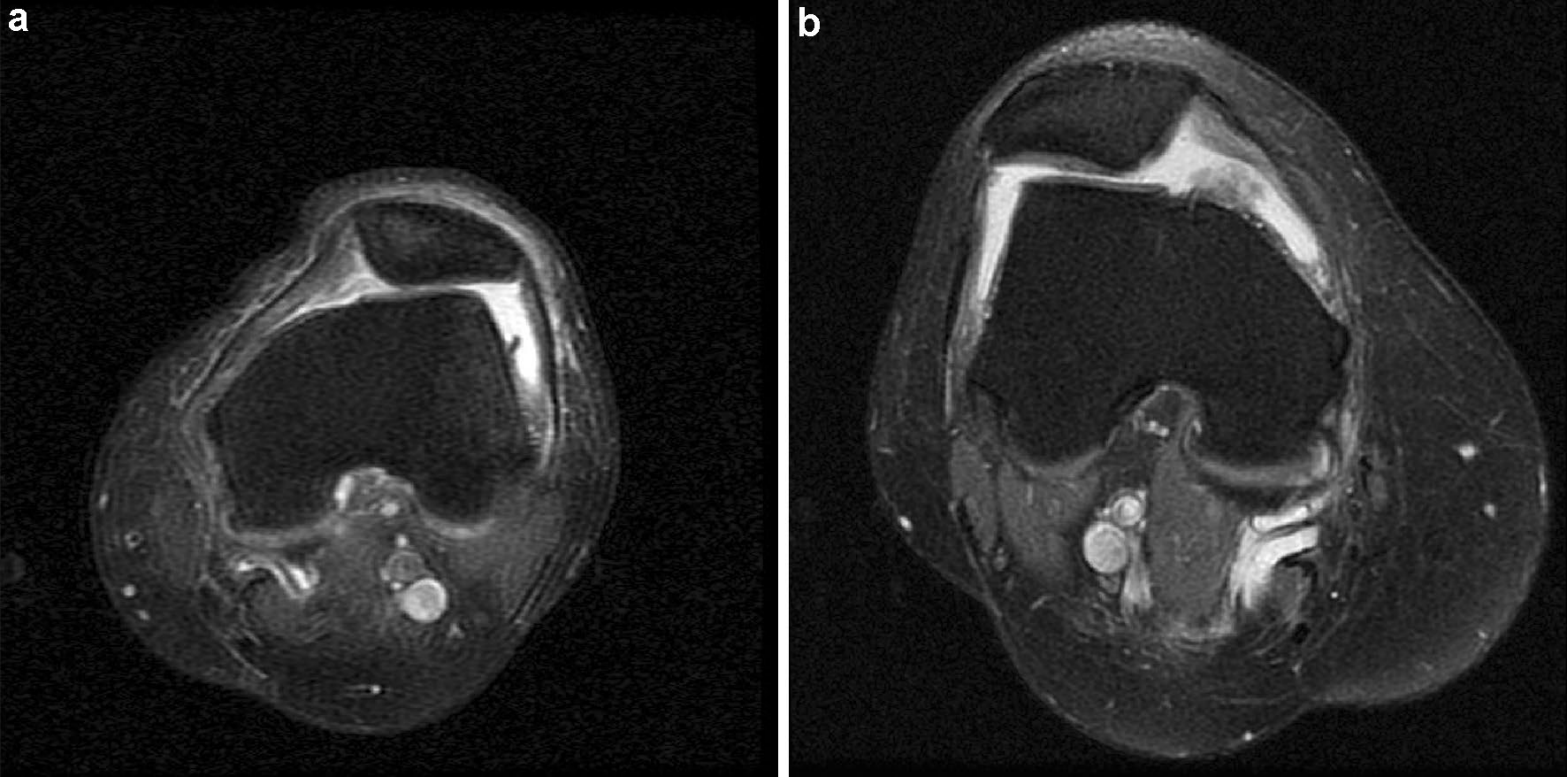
Axial PD-fatsat MRI (a section 3 cm above the knee joint). a Type B trochlear dysplasia is present. Note the flat trochlear groove and patellar subluxation. b Another patient was diagnosed with type B trochlear dysplasia, patellar subluxation, and patellar chondromalacia
